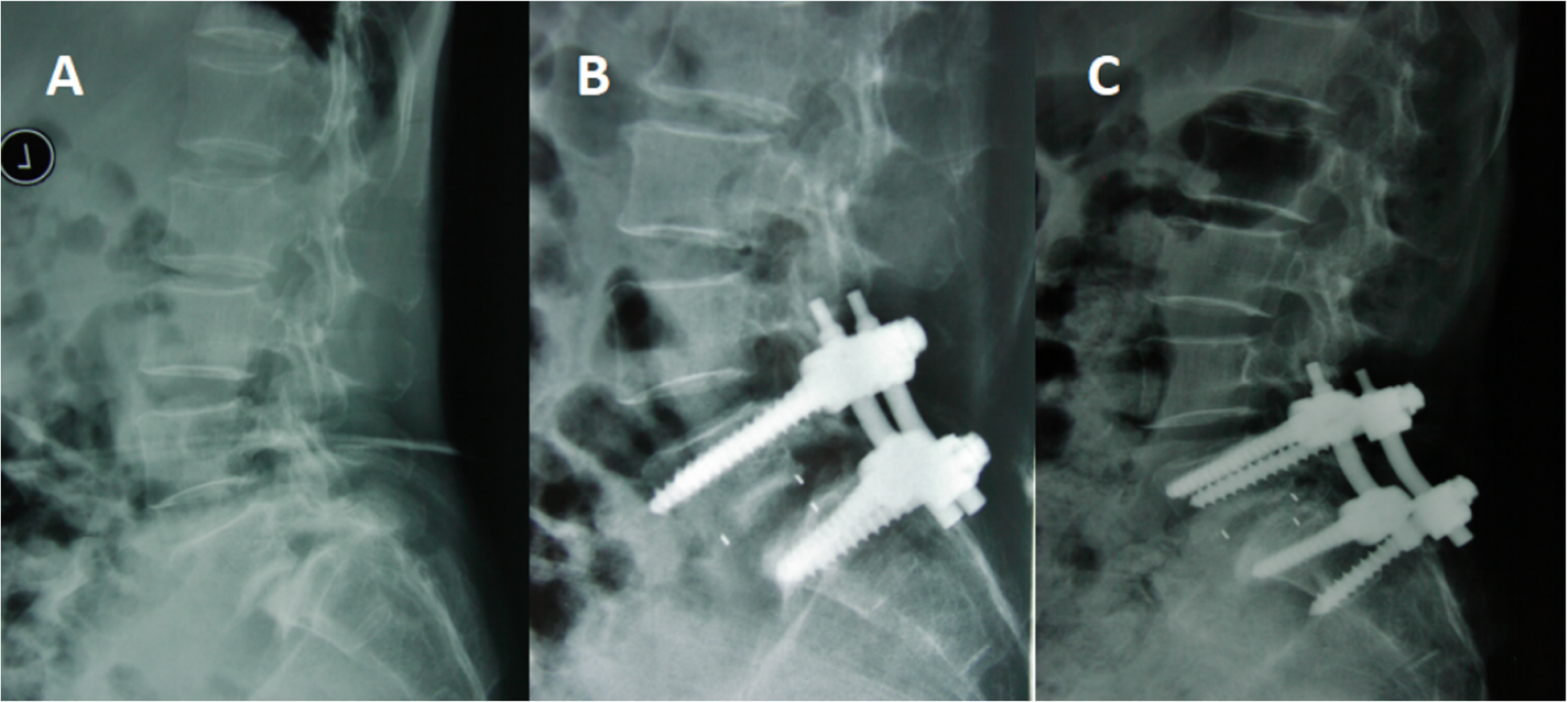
L5 DLS in a patient treated with L5 laminectomy and intervertebral interbody fusion. (a, b) Postoperative X-ray was normal. c The X-ray showed screw displacement and bone union at the 52-month follow-up
